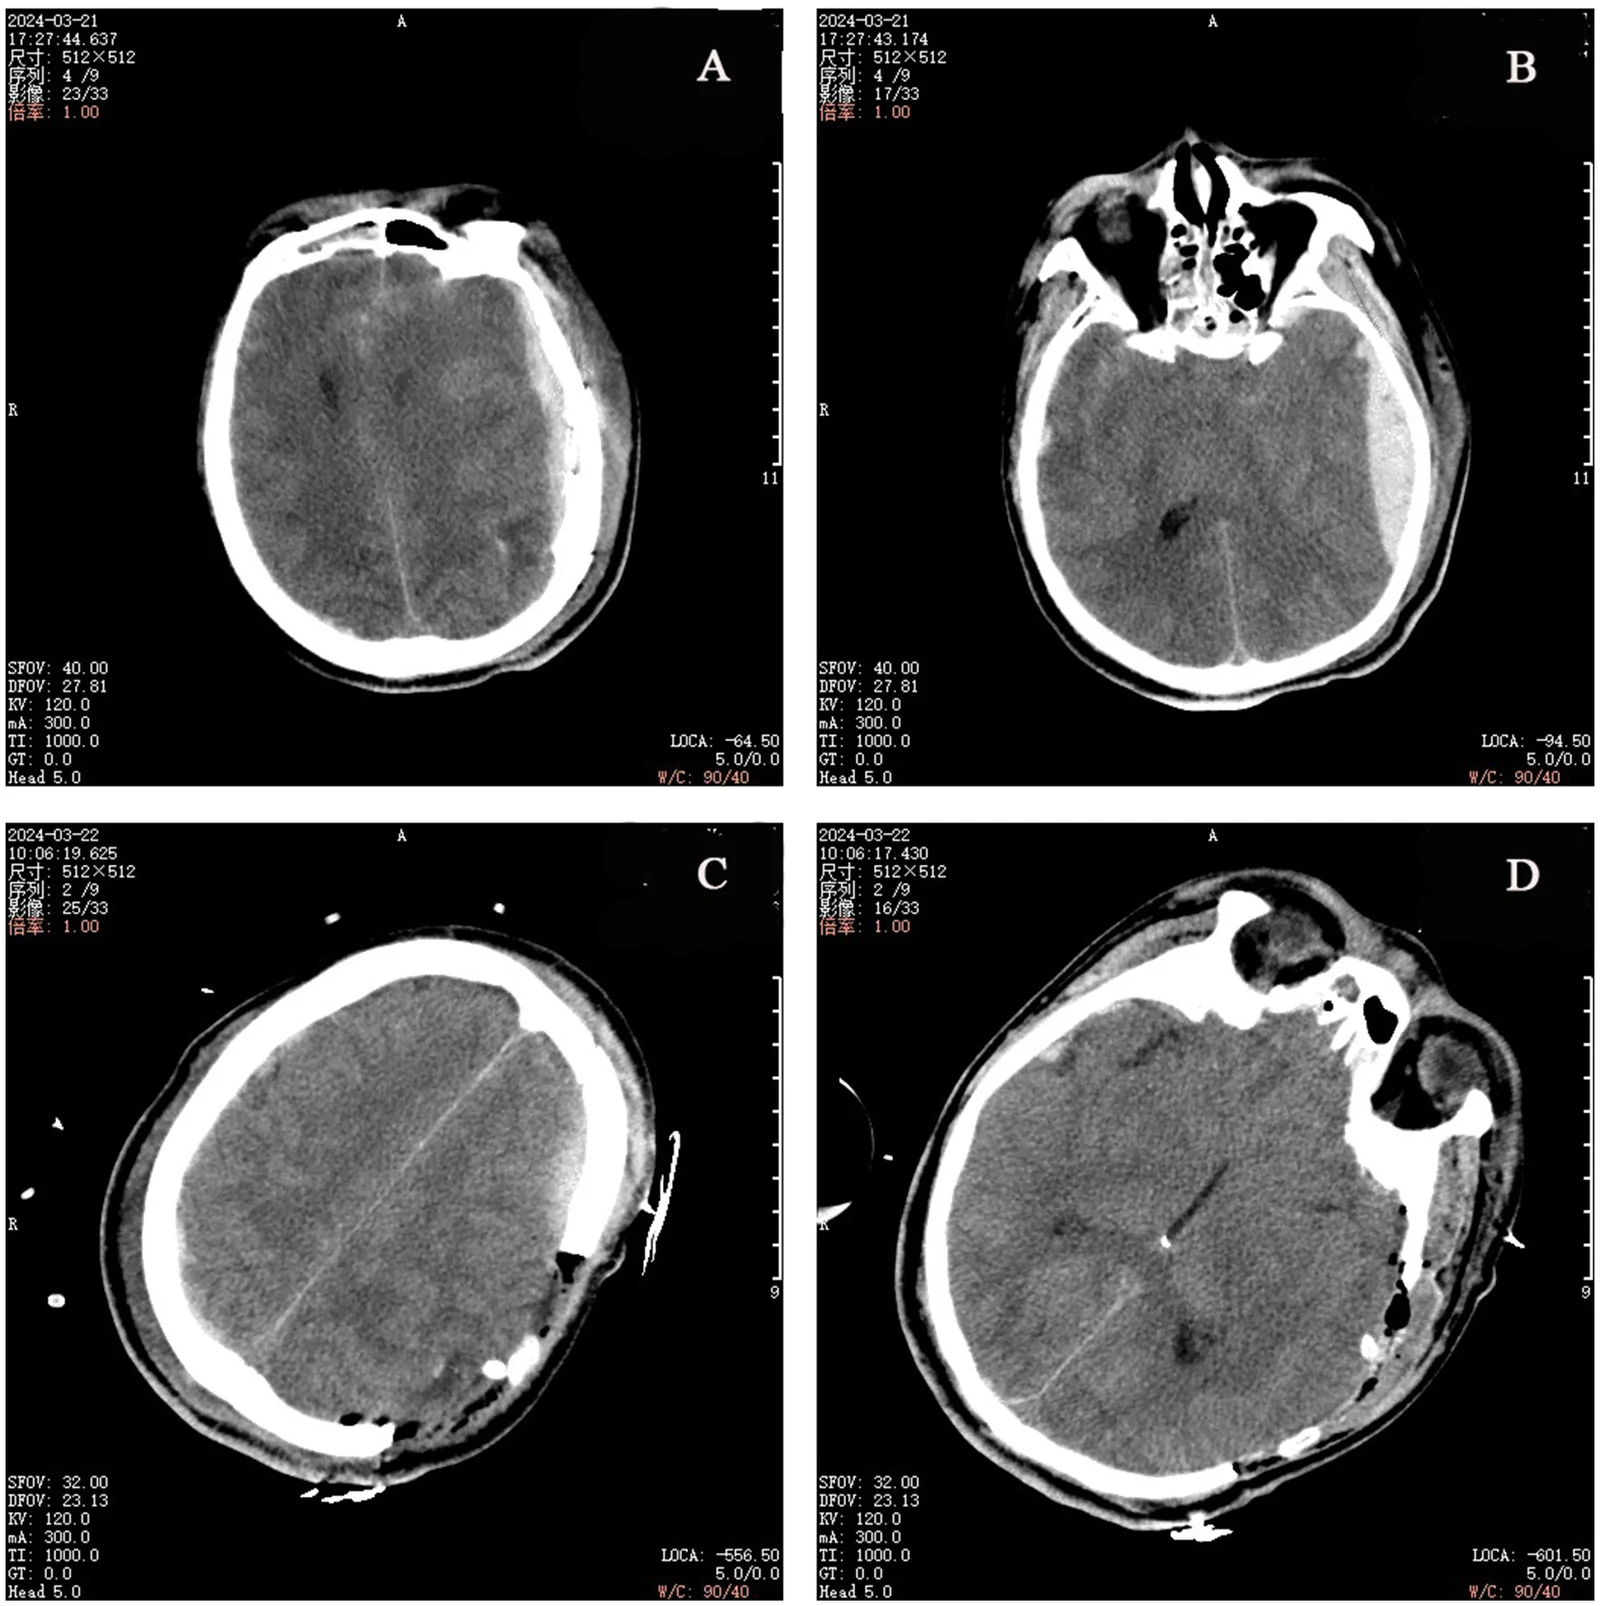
Preoperative and postoperative cranial CT images of Patient 1 with traumatic brain injury. (A, B) Preoperative CT scans showing midline shift, cerebral swelling, herniation, left temporal bone fracture, and cerebral contusion. Traumatic subdural and epidural hemorrhages are also evident. (C, D) Postoperative CT scans following decompressive craniectomy, demonstrating resolution of herniation and alleviated cerebral edema compared to preoperative findings.
